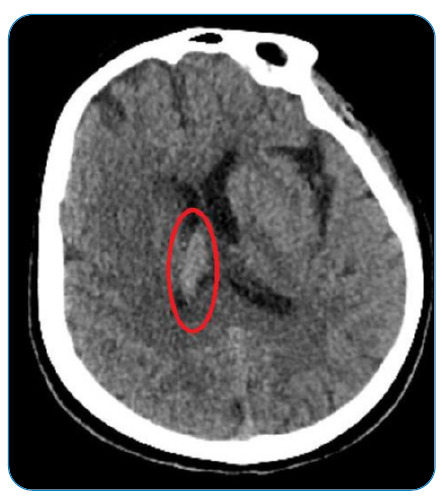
MRI of the brain showing more prominent bilaterally hyperintense areas adjacent to the right lateral ventricle in the FLAIR sequence. This was evaluated as being compatible with ventriculitis.
